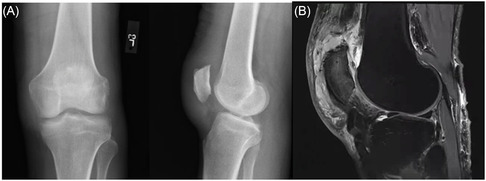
Left knee imaging 11 days after suture anchor quadriceps tendon repair following a fall. (A) A‐P and lateral radiographs show an effusion and recurrent patella baja with a Caton‐Deschamps index of 0.65. (B) Representative T2‐weighted sagittal MRI cut showing a complete retear of the distal quadriceps tendon. (MRI, magnetic resonance imaging; A‐P, anterior‐posterior.)
